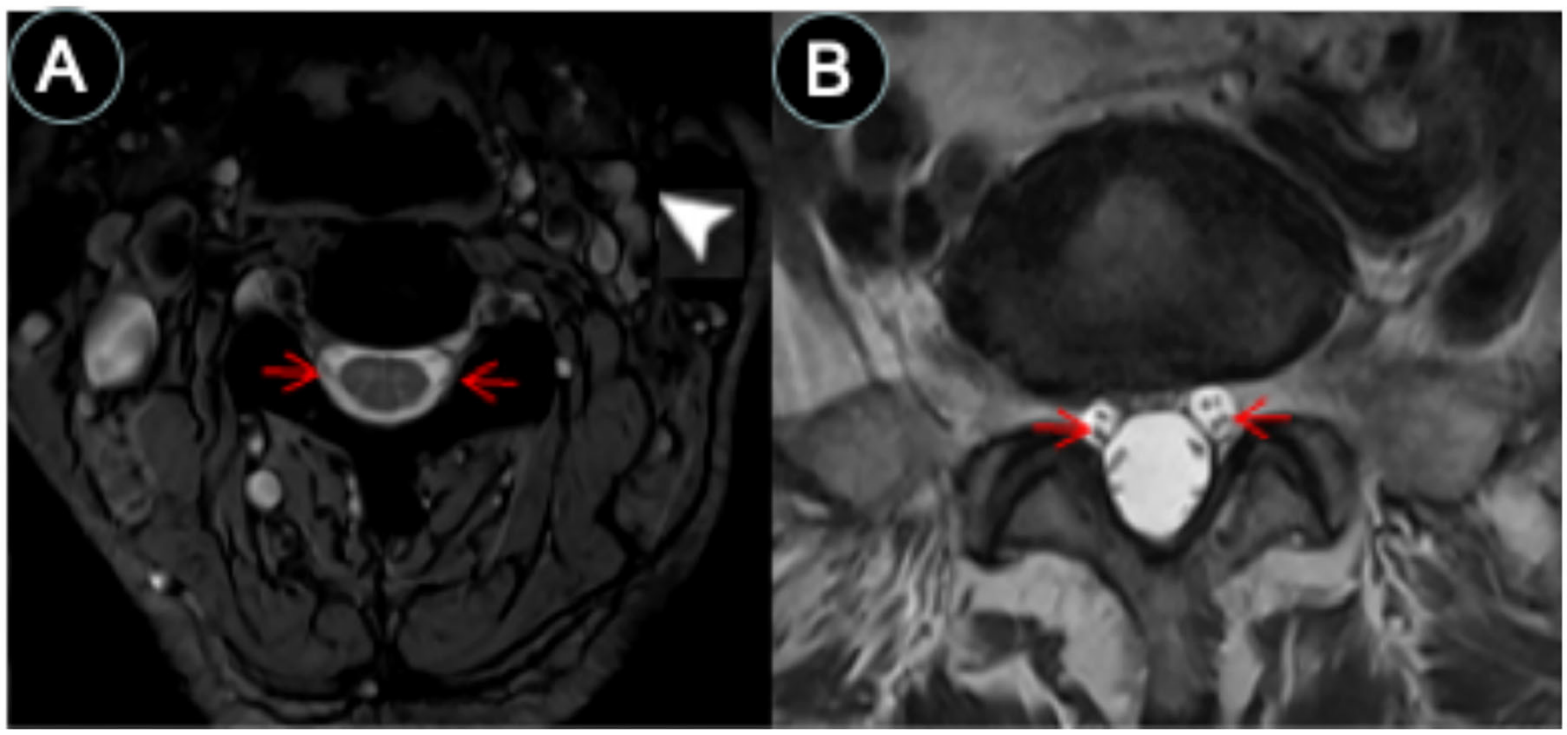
CSF-filled peri-neural spaces surrounding spinal nerves. (A) Cervical spinal nerves (brachial plexus). Cervical nerves four are pointed with red arrows (hypointense signal), and the space surrounding the nerves is the CSF-filled peri-neural space (hyperintense signal). Multiple lymph nodes are detected from MRI (white arrowhead). (B) Lumbar spinal nerves (red arrows, hypointense signal) surrounded with CSF-filled peri-neural space (hyperintense signal). Images were generated from the third and the fourth experiments as described in the method section.
